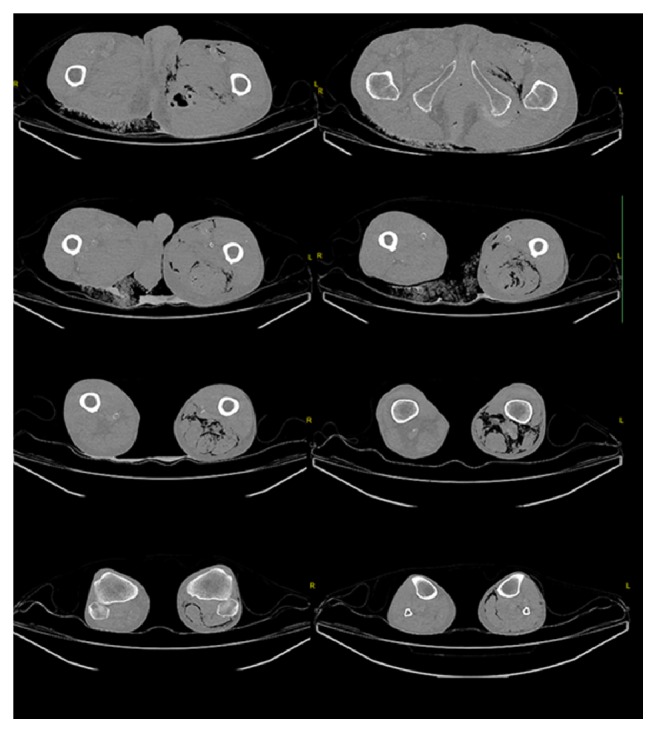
Axial CT scan of the lower leg starting from the hip to the knee with sharply demarcated free air in the soft tissue.
